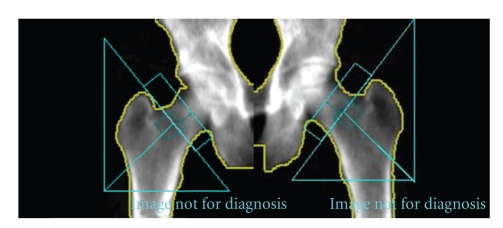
Left and right hip dual energy X-ray absorptiometry scans showing regions of interest used for analysis.
